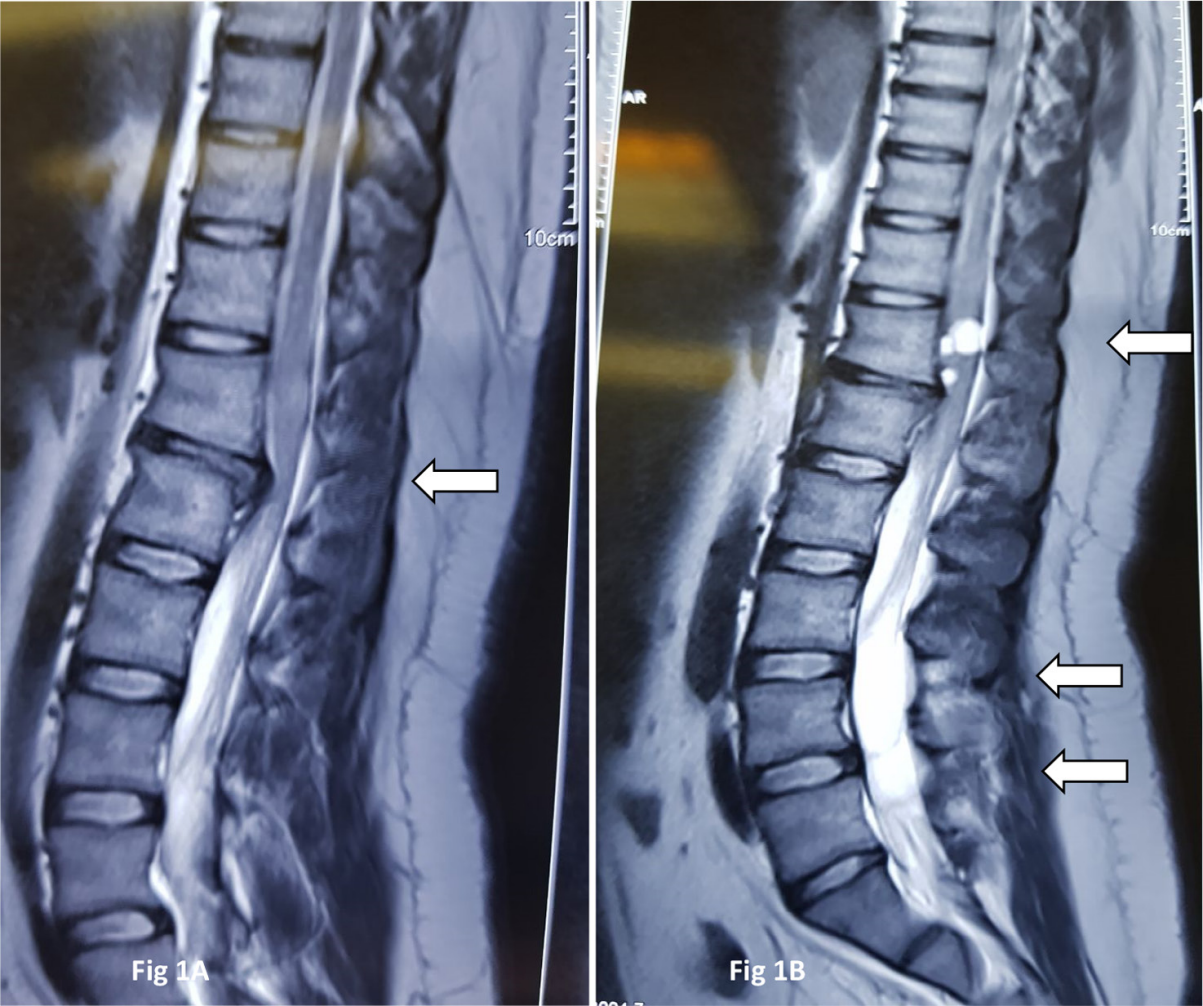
Pre‐operation imaging. (A) Sagittal T2‐weighted MRI shows extruded disc at the level of T12‐L1 (white arrow). (B) Sagittal T2‐weighted MRI shows three well‐demarcated cystic lesions with different diameters at the level of T12 vertebral body. Two other lesions with the same characteristics were present at the level of L4/L5 vertebral bodies (white arrows).
